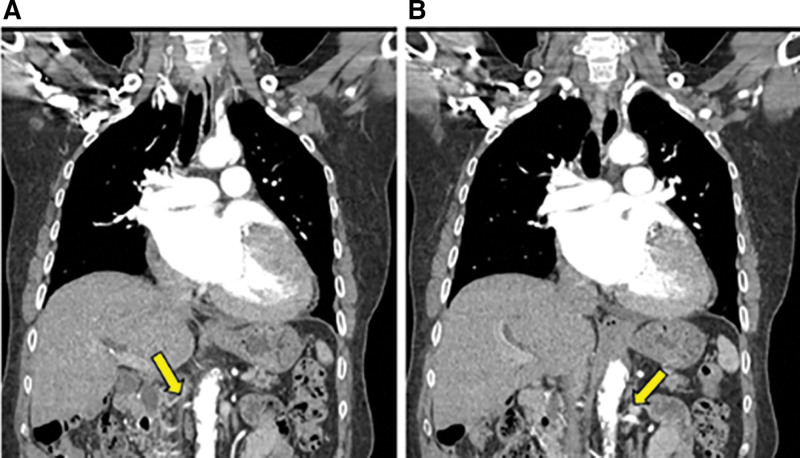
Chest computed tomography before coronary artery stent insertion. The orifice of both renal arteries shows stenosis. (A) Right kidney artery stenosis. (B) Left kidney artery stenosis.
